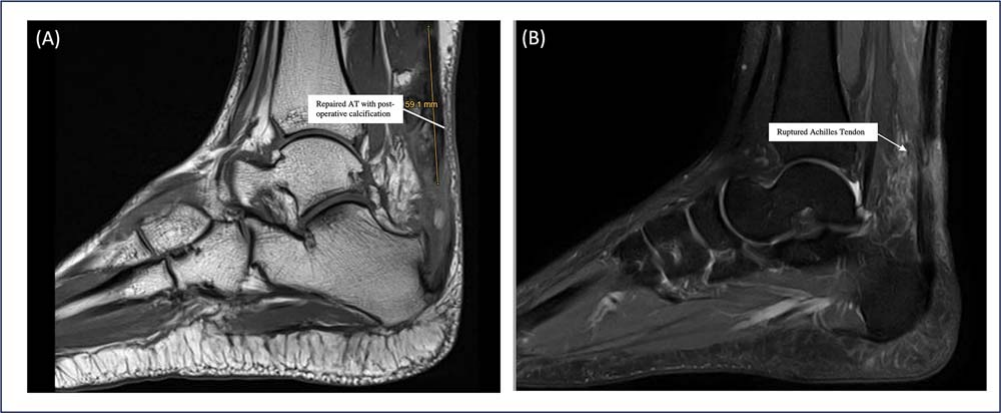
Sagittal MRI sequences of Patient 5 showing calcific changes and tendon status. (A) MRI highlights a 6 mm postoperative calcification near the Achilles tendon insertion site. (B) MRI confirms intact Achilles tendon continuity with no evidence of re-tear.
